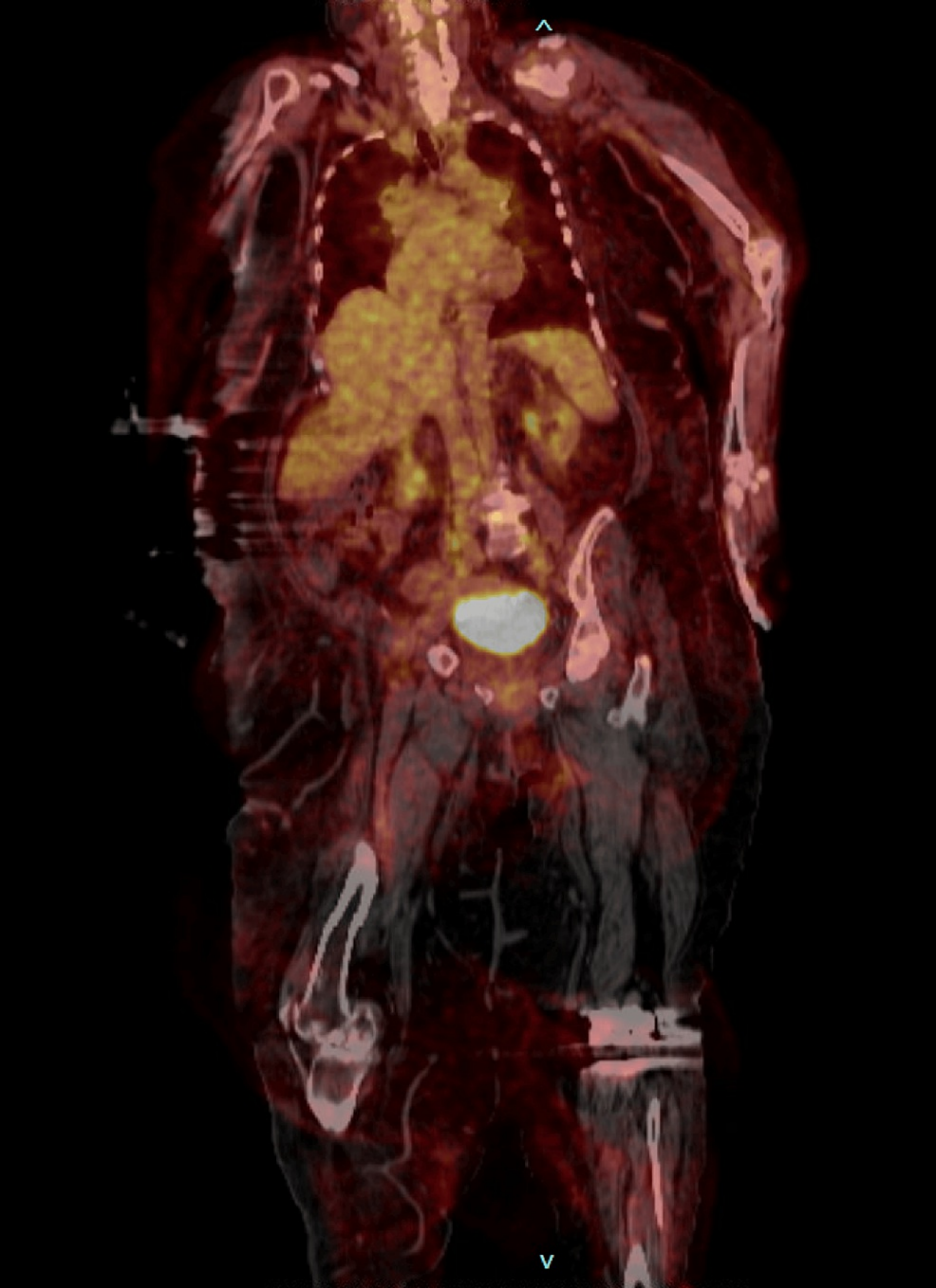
Positron emission tomography-computed tomography with no evidence of hypermetabolic activity.
